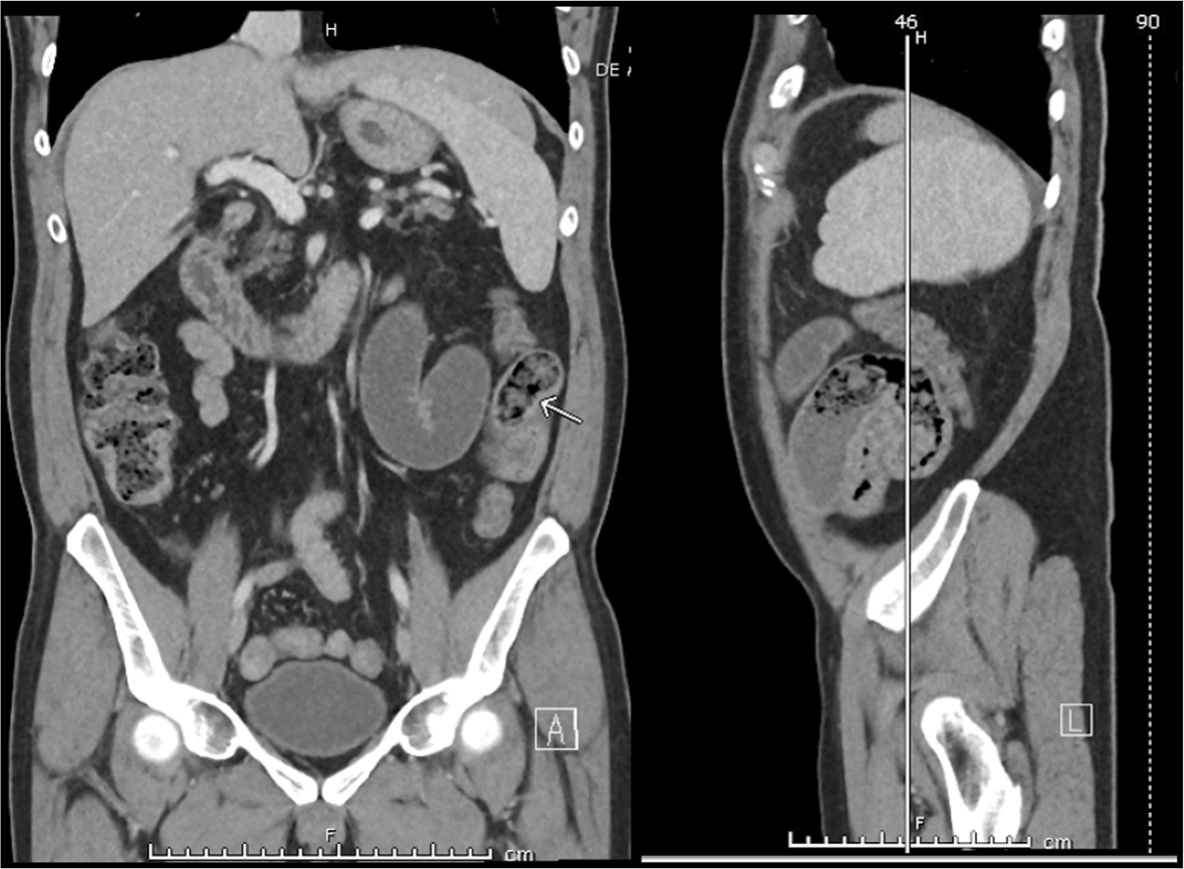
The small bowel feces sign. On further inspection, the patient’s CT scan was determined to have a characteristic small bowel feces sign in the mid-jejunum, just proximal to the suspected transition point. Defined in 1995 as the presence of gas and particulate material in a dilated segment of the small bowel, the small bowel feces sign indicates fecal content in the small bowel and is due to intraluminal stagnation of enteric material [11].
